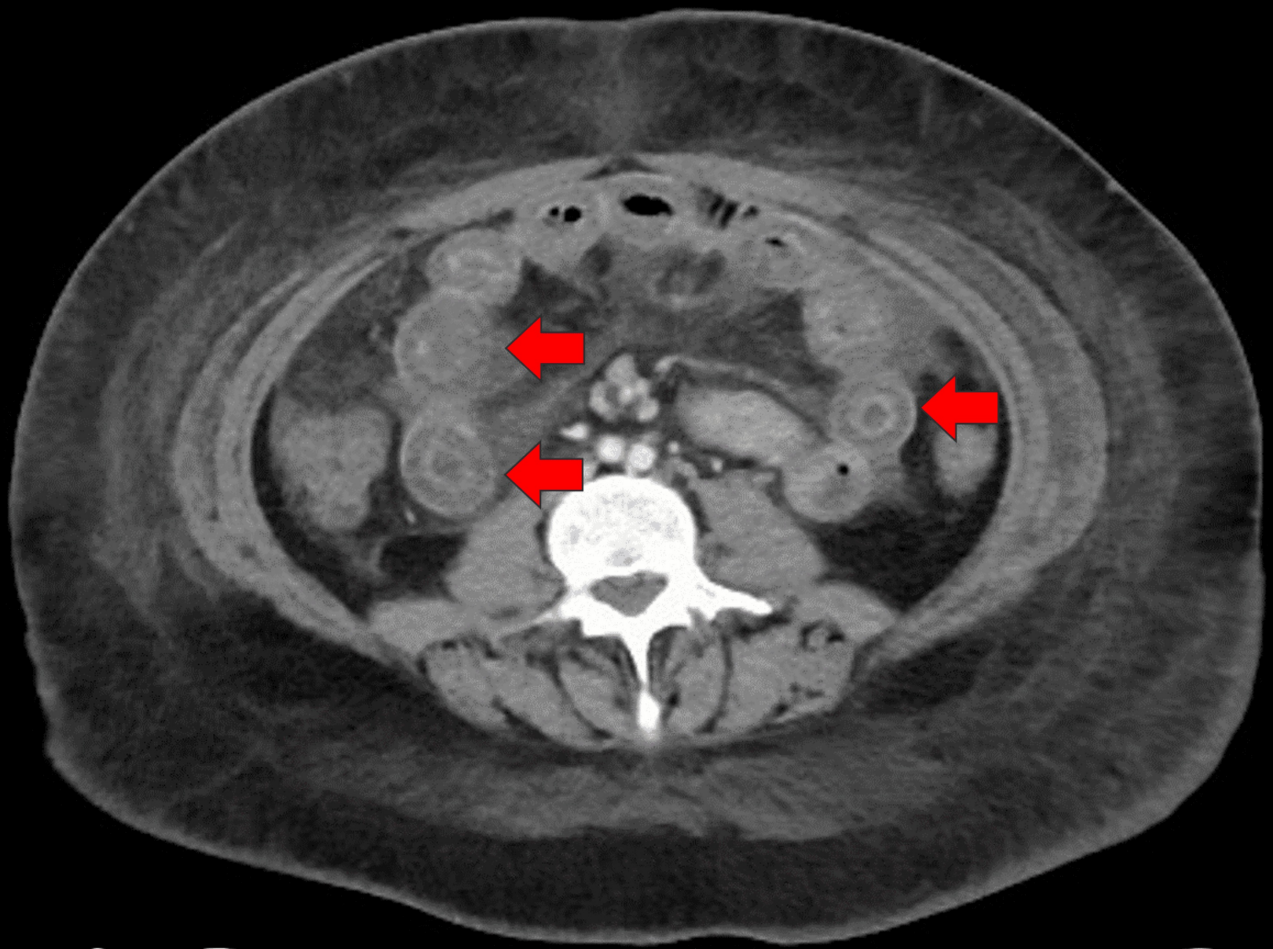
Abdominal CT scan (axial view) Small bowel loops showed diffuse edematous wall thickening with target signs (red arrows).
